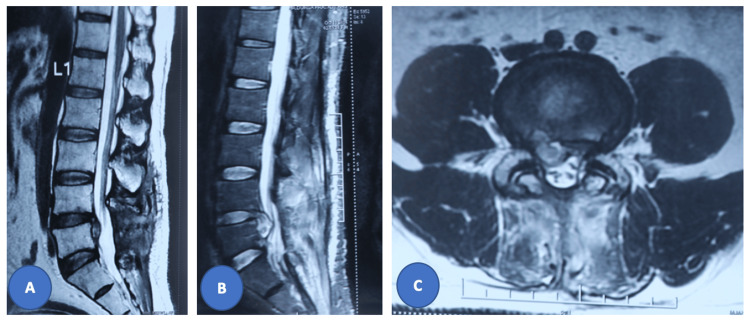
Preoperative MRI images of the index surgery showing right paracentral disc extrusion and caudal extension at the L4-5 disc causing severe canal stenosis. (A) T1 images of the sagittal section; (B) T2 images of the sagittal section; (C) axial images at the L4-5 level.
